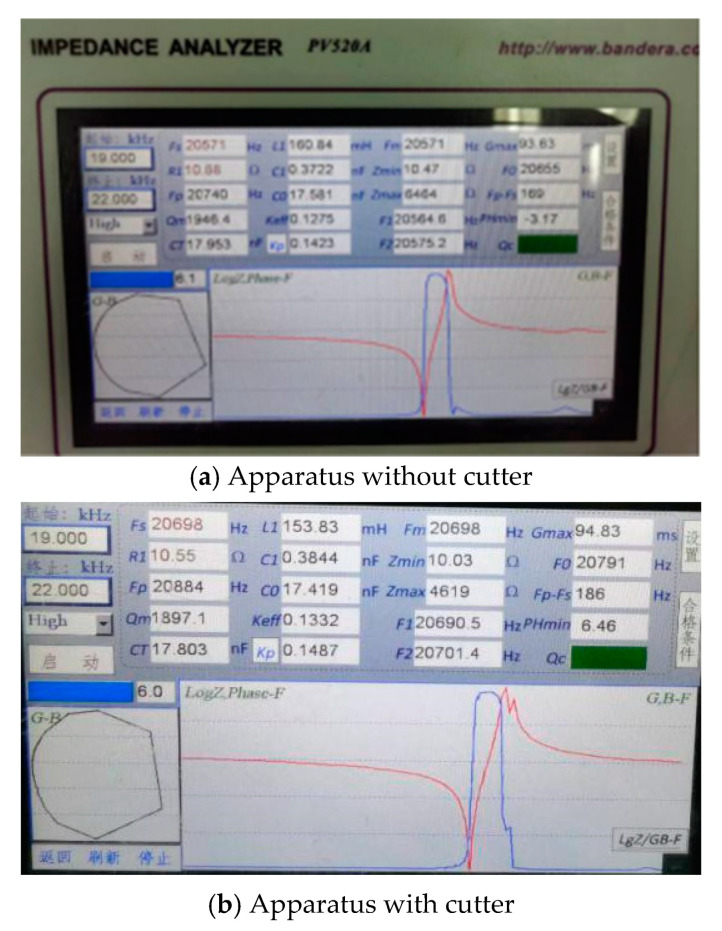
The testing origin data of ultrasonic vibration apparatus.
